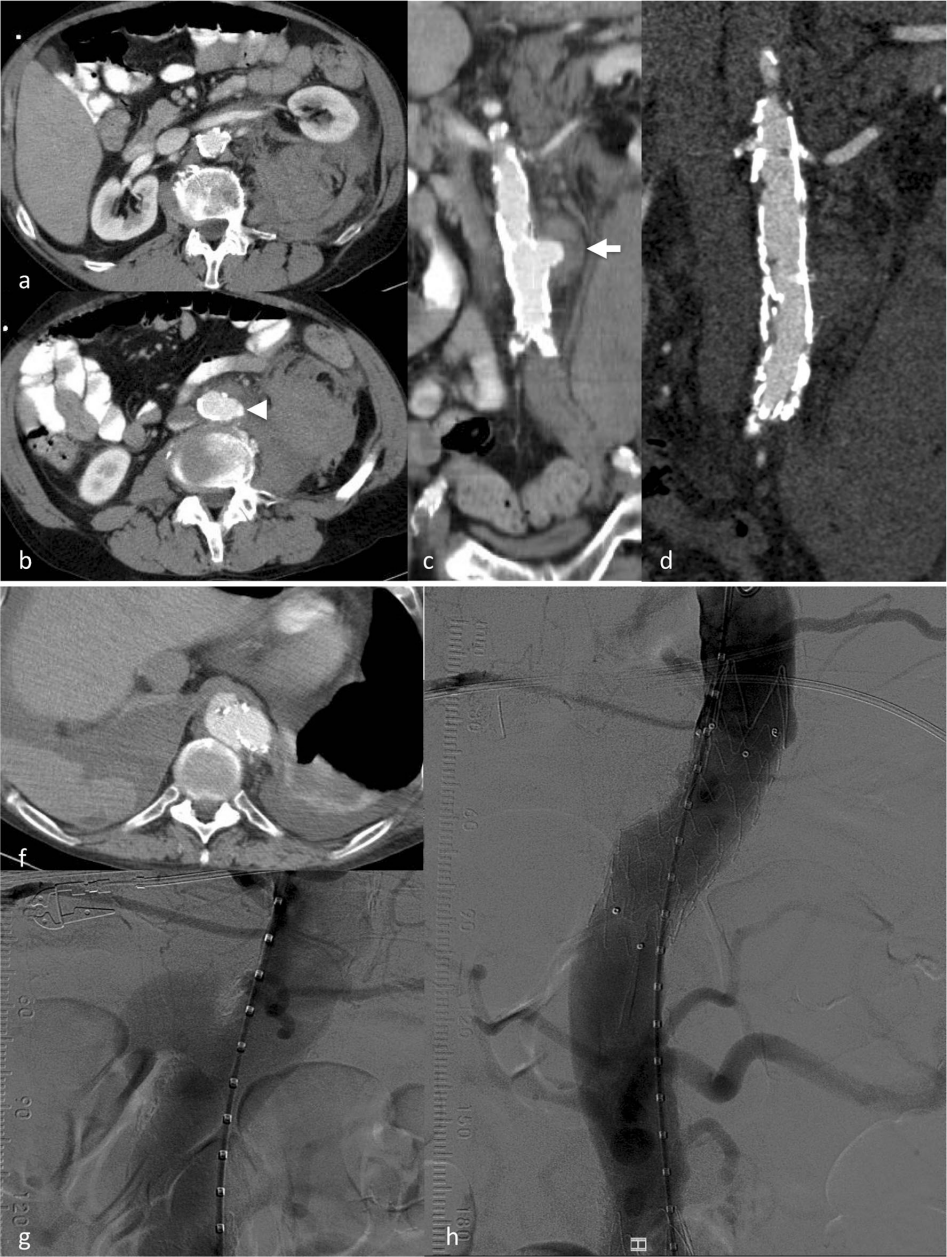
Ruptured penetrating aortic ulcer in a 57-year-old male patient. Chronic renal failure led to haemodialysis. The patient presented with hypotension and abdominal pain. CTA demonstrated a large retroperitoneal haematoma (a) and an aortic ulcer projecting beyond the aortic contour (*arrowhead*) (b). Coronal reformation demonstrated the mushroom-like appearance of the ulcer (*arrow*) (c). The patient was treated with implantation of a uni-iliac stent graft (d). Three years later he presented with hypotension and thoracic pain. CTA demonstrated a ruptured aortic ulcer in the distal descending aorta (e) that was again treated with implantation of a thoracic stent graft (f-g)
